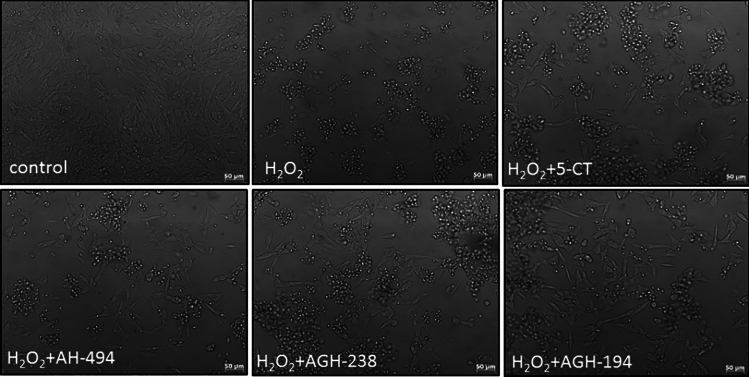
DIC (Differential Contrast Images) microphotographs of UN-SH-SY5Y cells cultured in NB experimental medium and treated with 5-HT_7_ agonists and H_2_O_2_. Cells were pre-treated for 30 min with 5-CT, AH-494, AGH-238 and AGH-194 at concentration of 0.1 µM followed by 24 h of treatment with H_2_O_2_ (150 µM)
